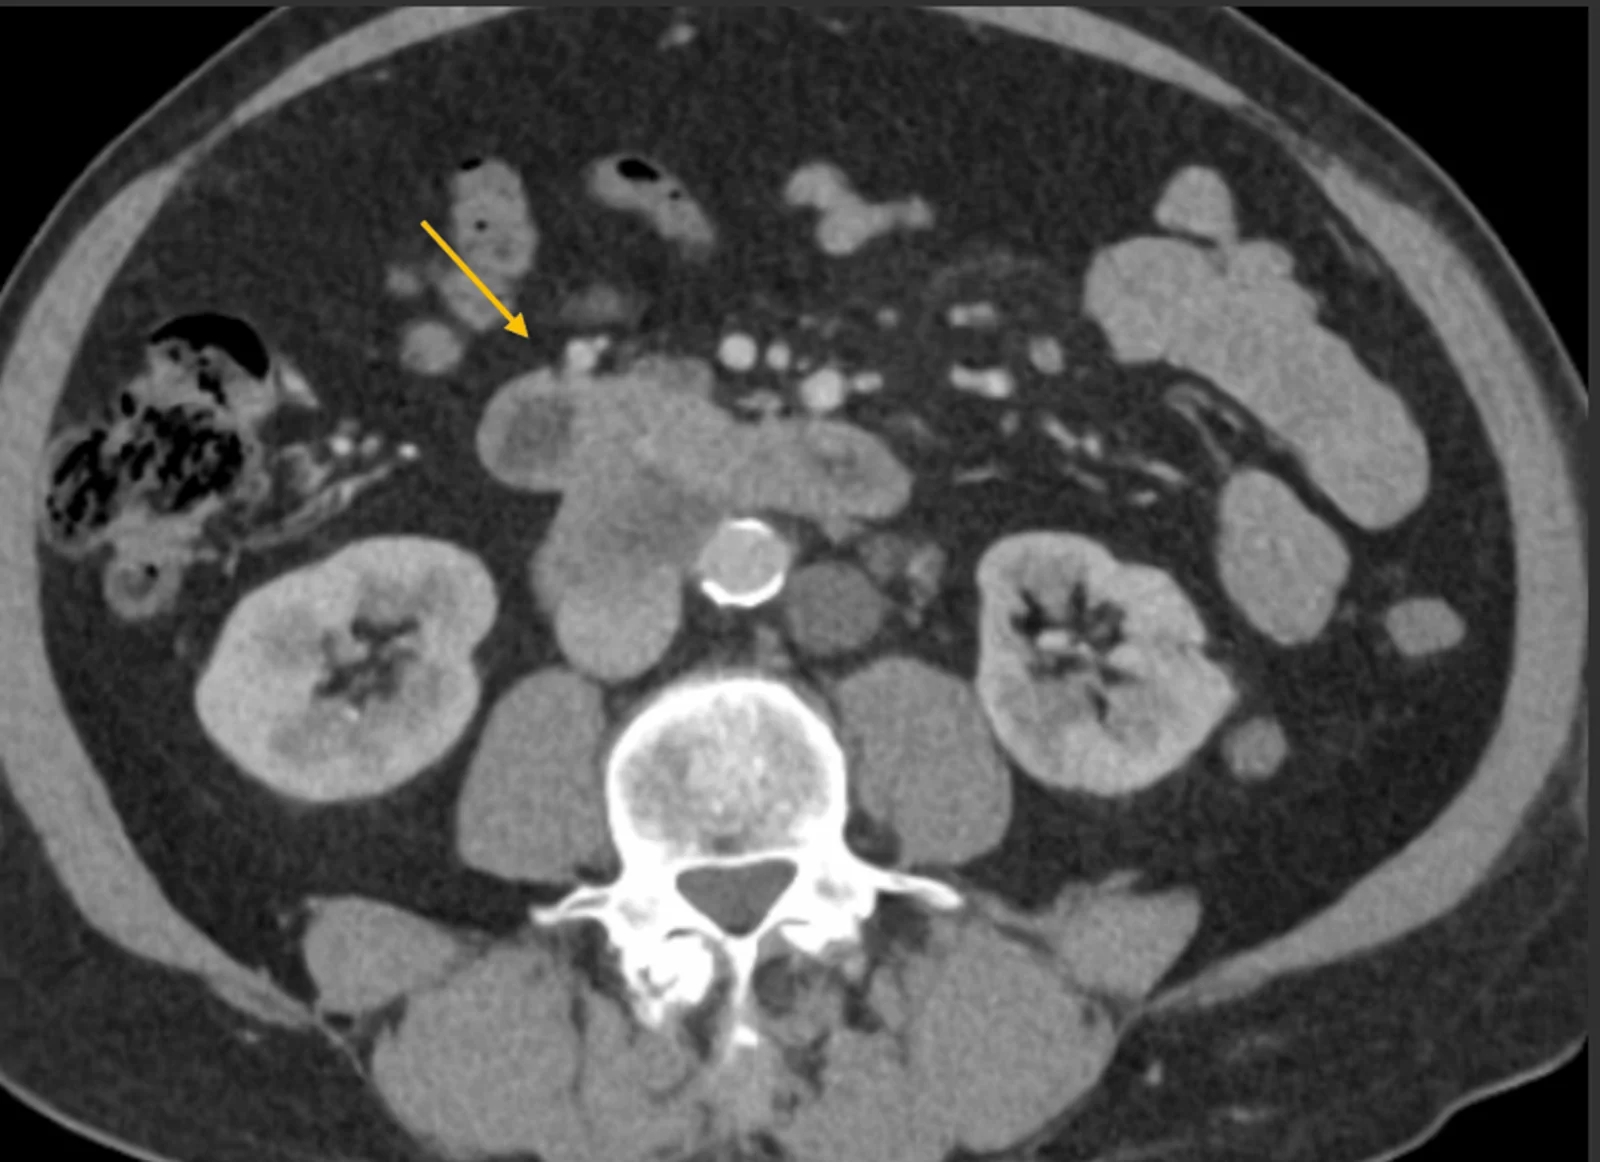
Contrast-enhanced axial CT image of the abdomen demonstrating progressive aortocaval lymphadenopathy (yellow arrow).
